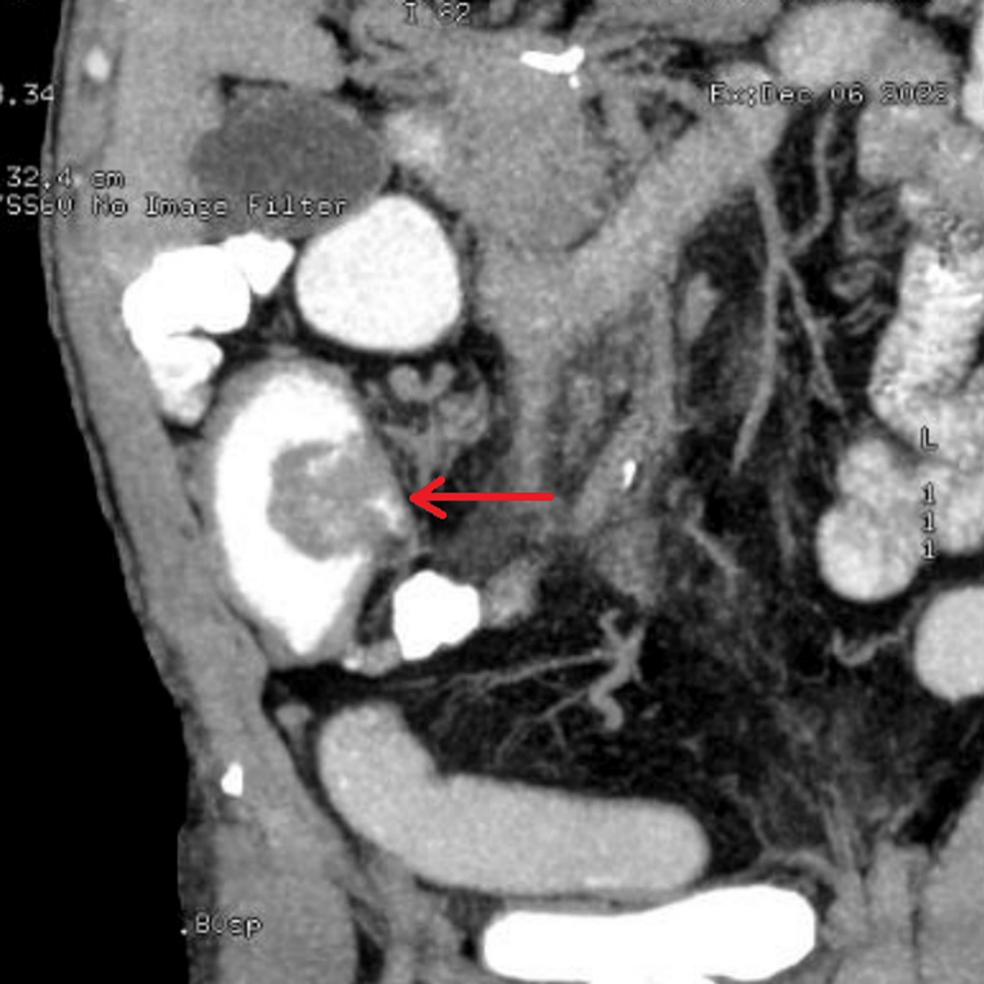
CT abdomen and pelvis with a red arrow pointing to a well-defined homogenously enhanced soft tissue mass at the ileocecal junction
